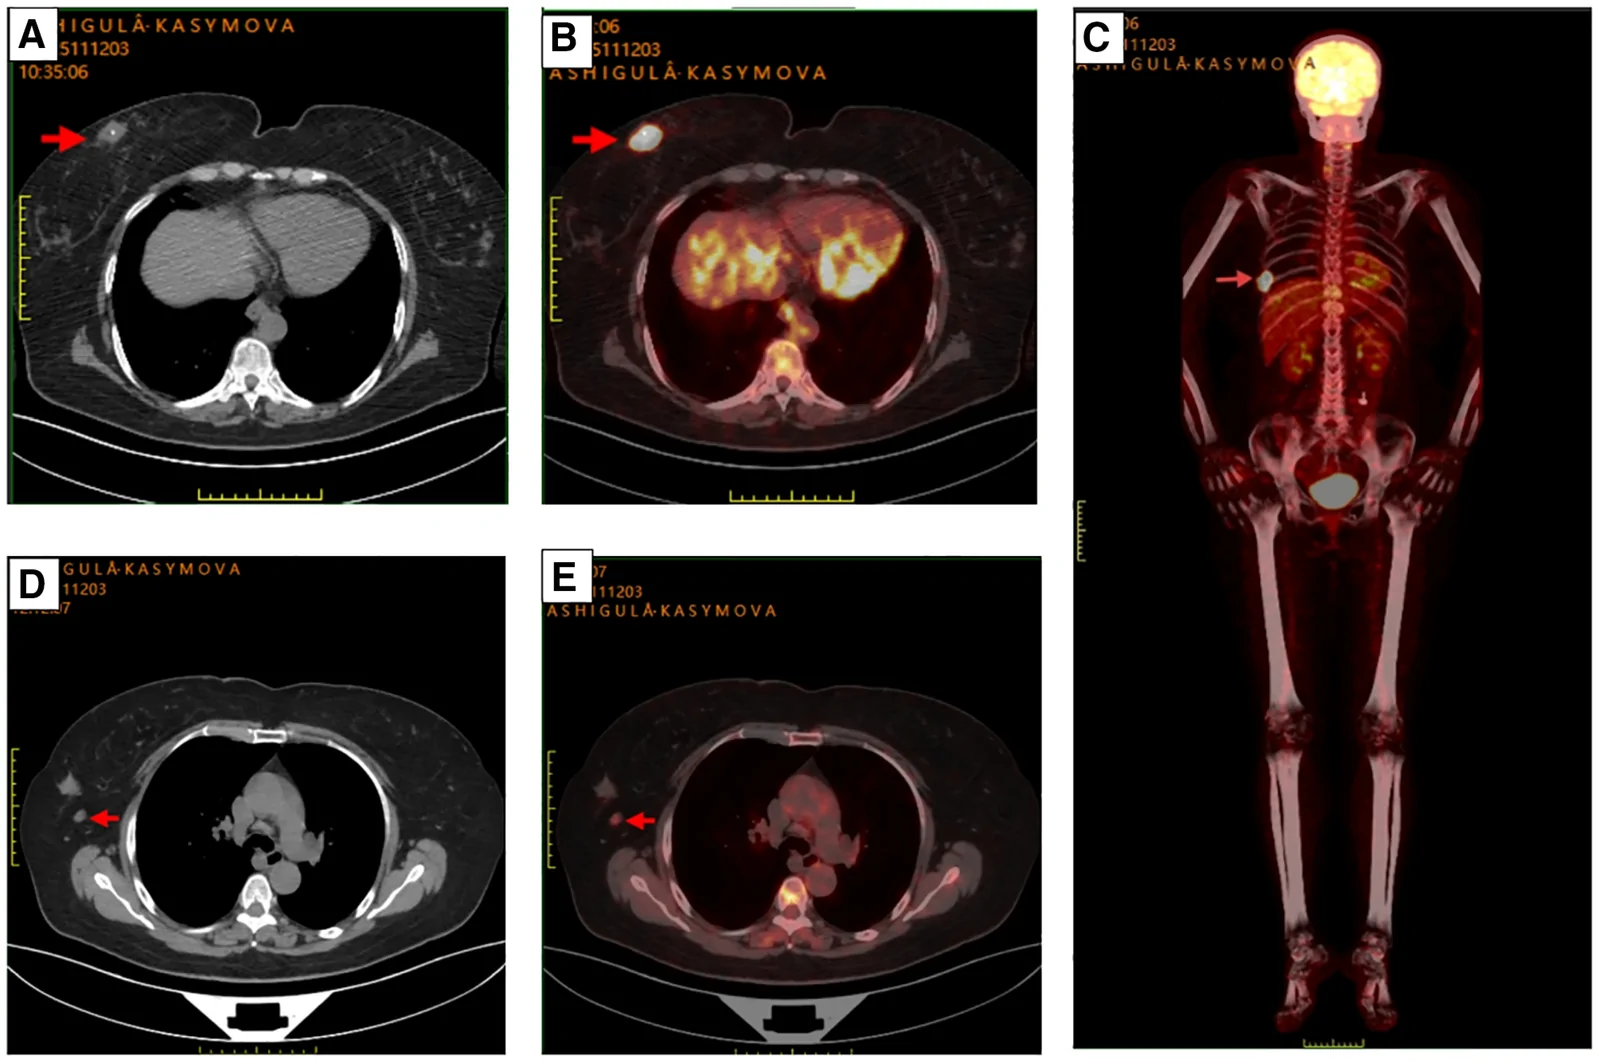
Positron emission tomography/computed tomography (PET/CT) findings. (A) PET/CT showed an isodense solid nodule with punctate calcifications in the right breast (arrow). (B, C) Markedly increased ^18F-fluorodeoxyglucose (^18F-FDG) uptake was observed in the lesion (arrows). (D) Enlarged lymph nodes in the right axilla (1.1 × 1.0 cm, arrow). (E) Enlarged right axillary lymph nodes showed mildly increased metabolic activity (arrow). PET/CT = positron emission tomography/computed tomography; ^18F-FDG = ^18F-fluorodeoxyglucose
